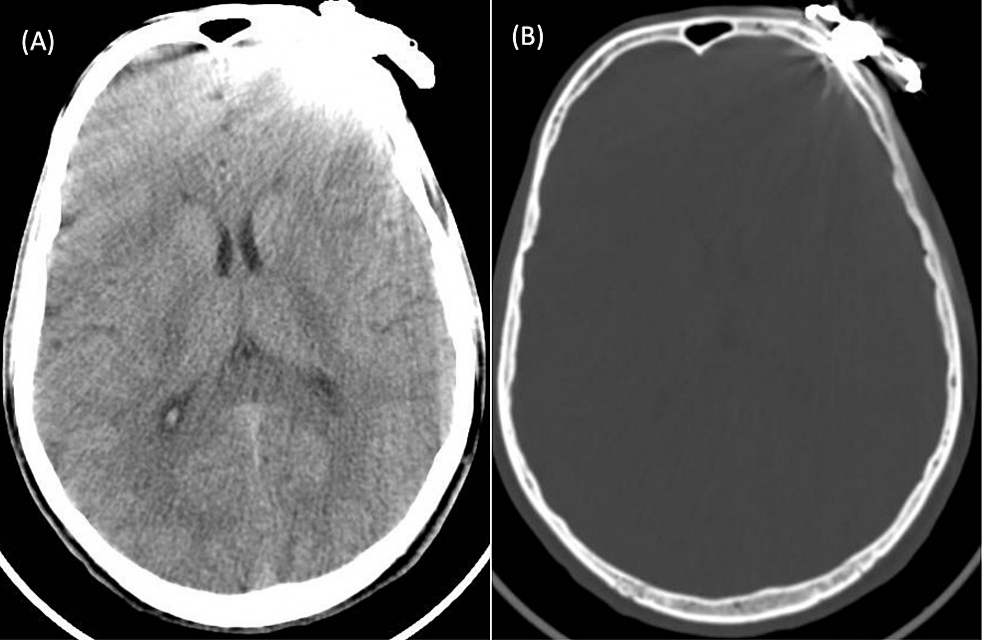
Computed tomography (CT) of the head. (A) Axial view of the brain showing the metal object on the left frontal bone with artifact effect. (B) Axial view of the brain (bone window) showing the metal object minimally embedded in the external table of the frontal bone.
